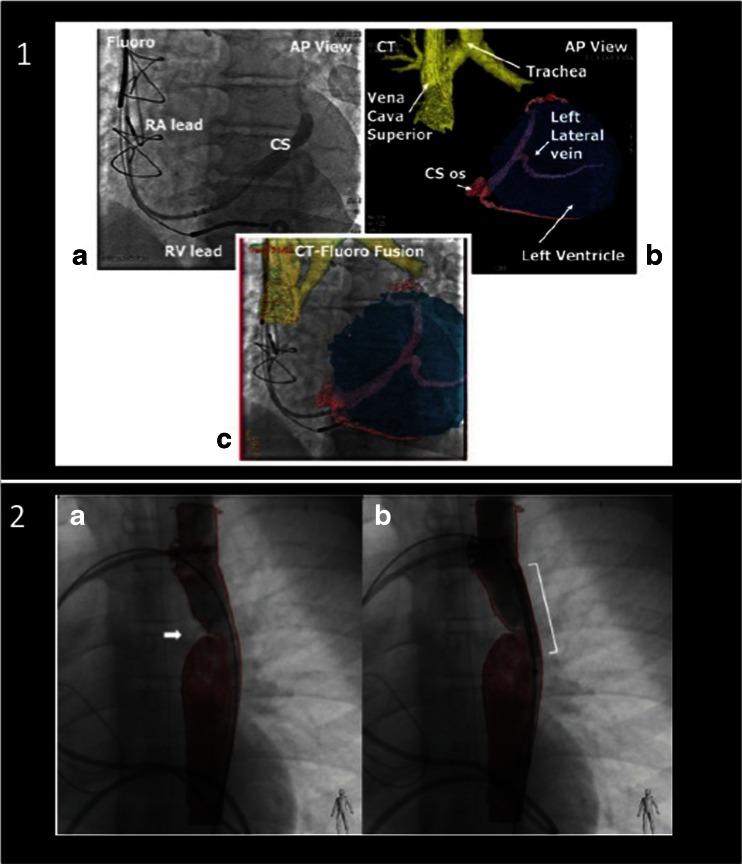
Fusion of computed tomography with fluoroscopy. Fusion of CT and fluoroscopy images has been used for interventions such as implantation of left ventricular leads for cardiac resynchronization (*1*, a–c) or for planning interventions in congenital heart disease such as coarctation of the aorta (*arrow* in *2*, *a* and *b*). *CS*, coronary sinus; *RV*, right ventricle; *RA*, right atrium. Reprinted from [19•, 30] with permission
